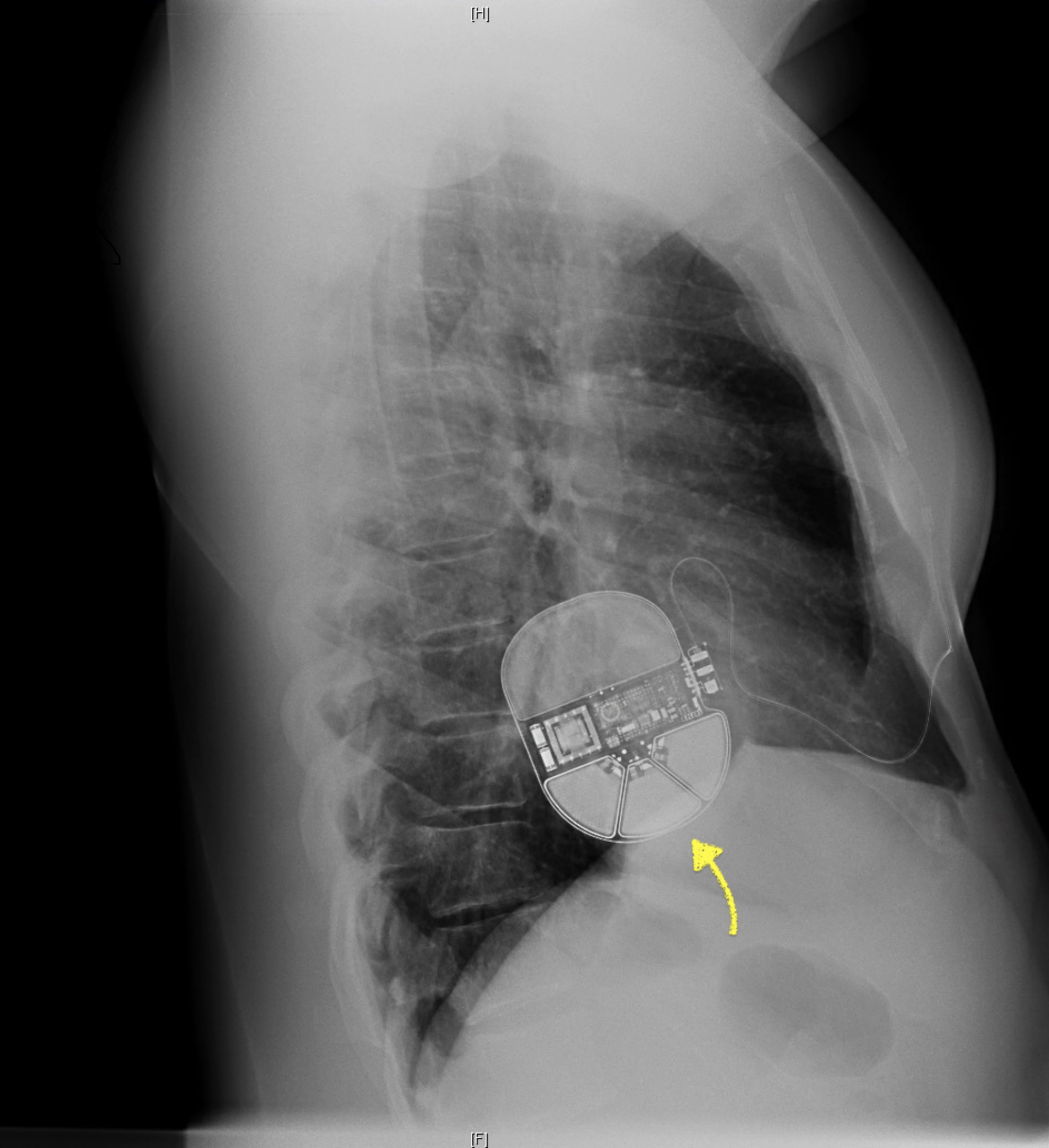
Automatic implantable cardioverter-defibrillator (AICD) Lateral view of chest X-ray showed intact left-sided AICD without evidence of active cardiopulmonary disease processes. H: head. F: foot.
